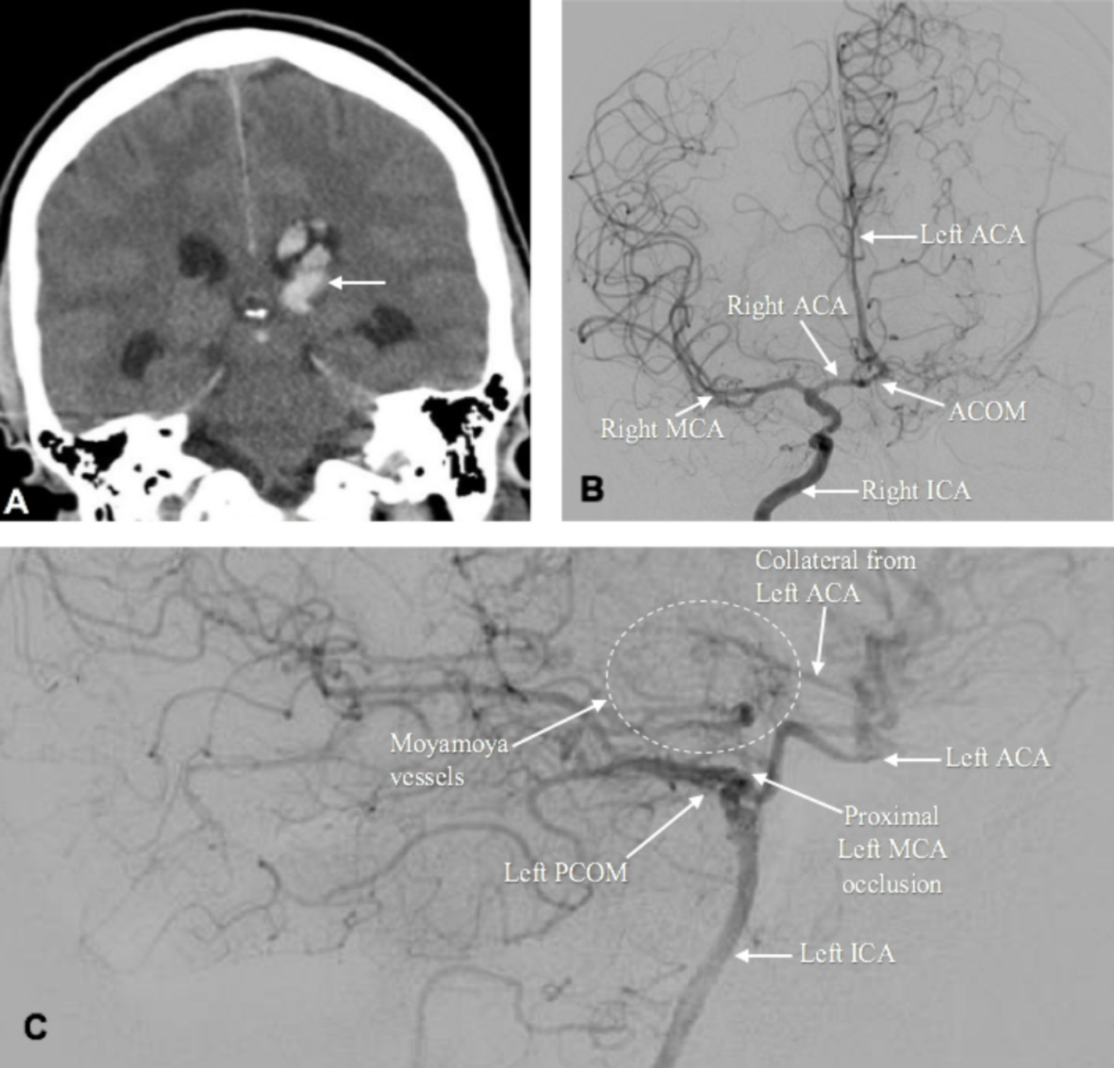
Panel A: noncontrast head CT showing rupture of the left thalamic hematoma into the lateral ventricle; Panel B: cerebral angiogram A-P view demonstrating right ICA injection showing the right ICA supplying the left ACA via the ACOM, as well as moderate right ICA stenosis; Panel C: cerebral angiogram lateral view demonstrating left ICA injection showing occlusion of the left ICA, as well as the left MCA territory being collateralized through leptomeningeal collaterals from the left ACA, which is supplied by the right ICA via the ACOM. Moyamoya vessels are present in the left basal ganglia area, left thalamic area, and left ethmoidal area. ICA, internal carotid artery; ACA, anterior cerebral artery; MCA, middle cerebral artery; ACOM, anterior communicating artery; PCOM, posterior communicating artery.
